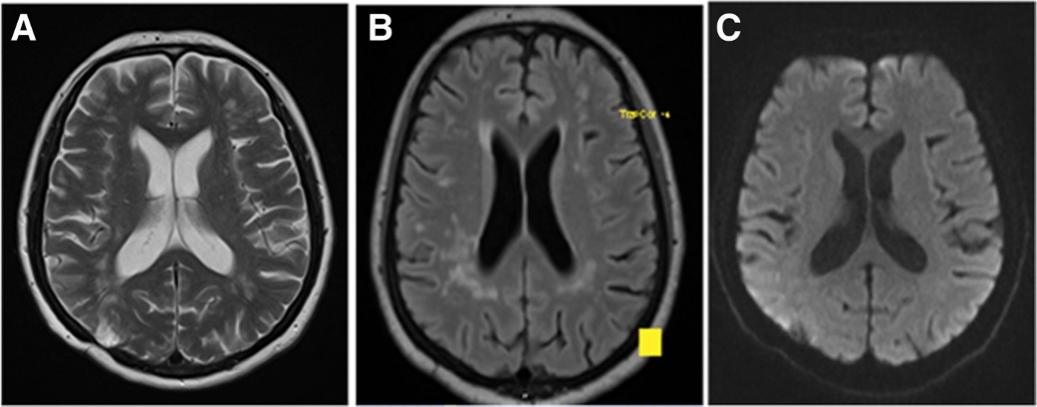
MRI-scan at 1-year follow-up visit. Markedly reduced white matter lesions. Right occipital hyperintensity on T2 reflects brain biopsy area. A) T2, B) T2-FLAIR, C) DWI.
